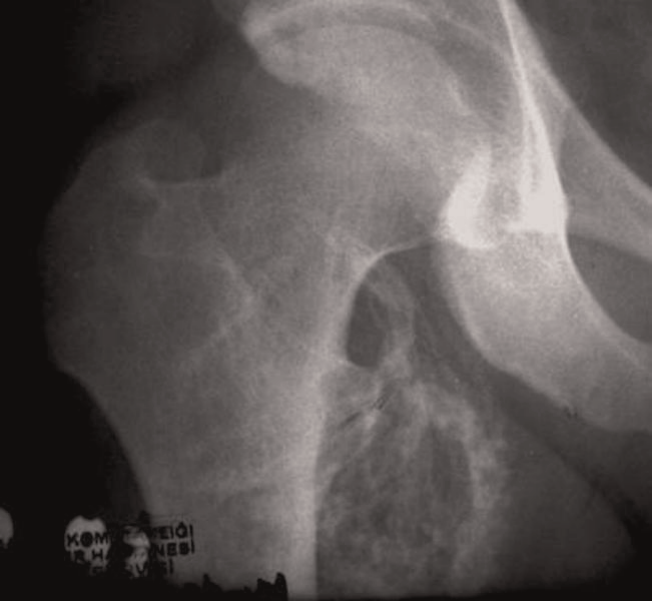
Direct radiography showing dispersed soft tissue calcifications.
